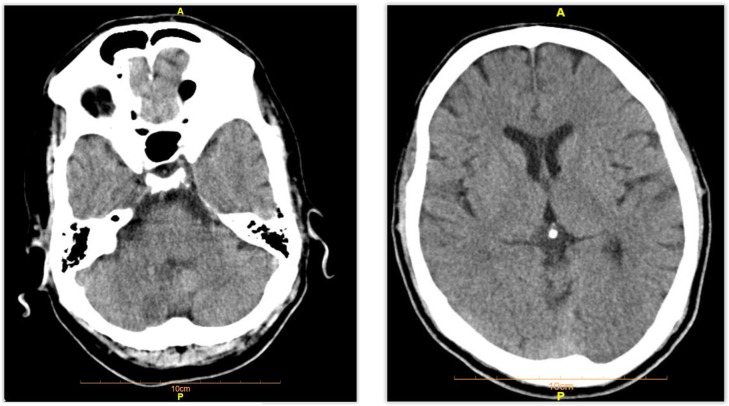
Axial Brain CT scan of the patient.
